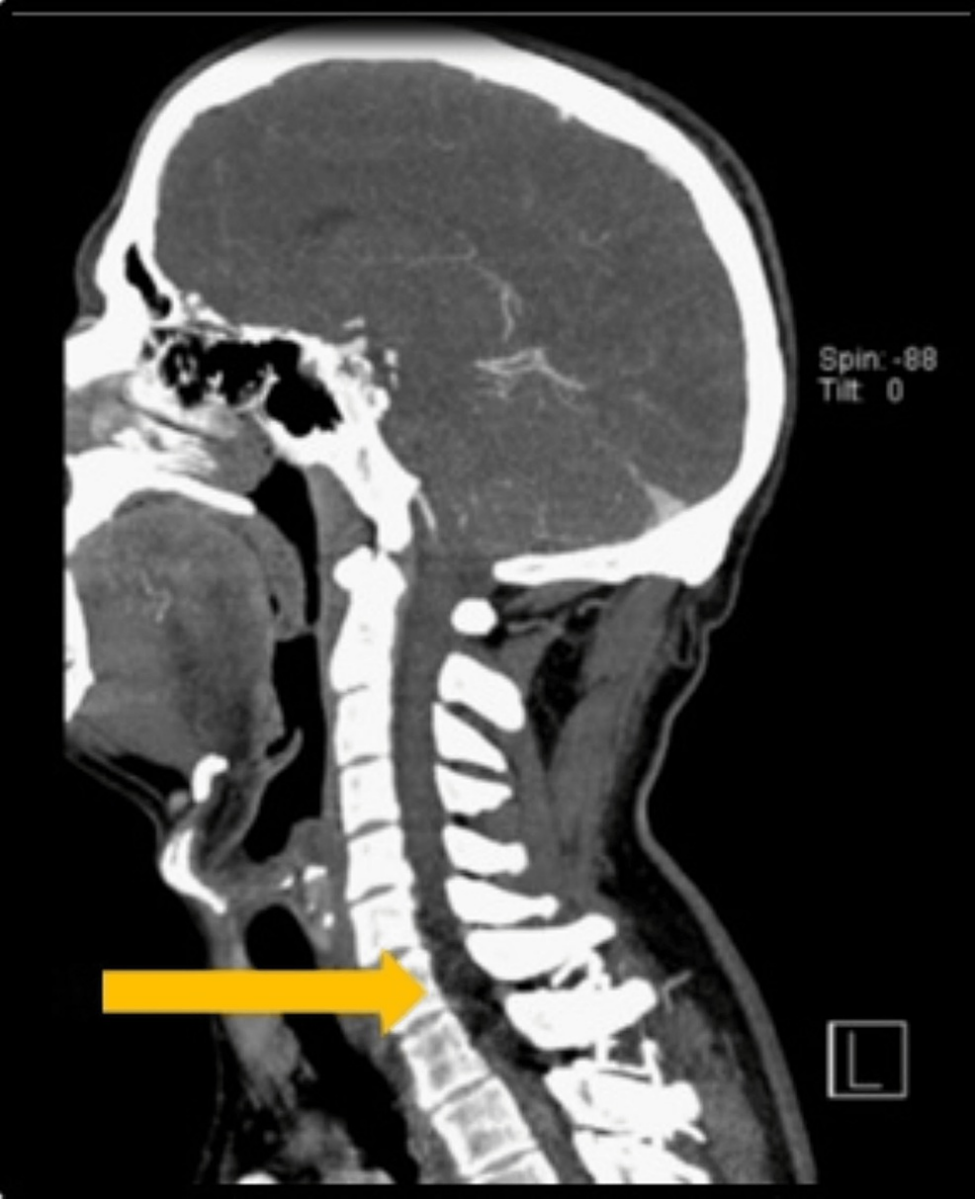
Computed tomography angiography scan showing the lesional area on sagittal view. An arrow pointing to the lesional area extending from C5 to T2.
